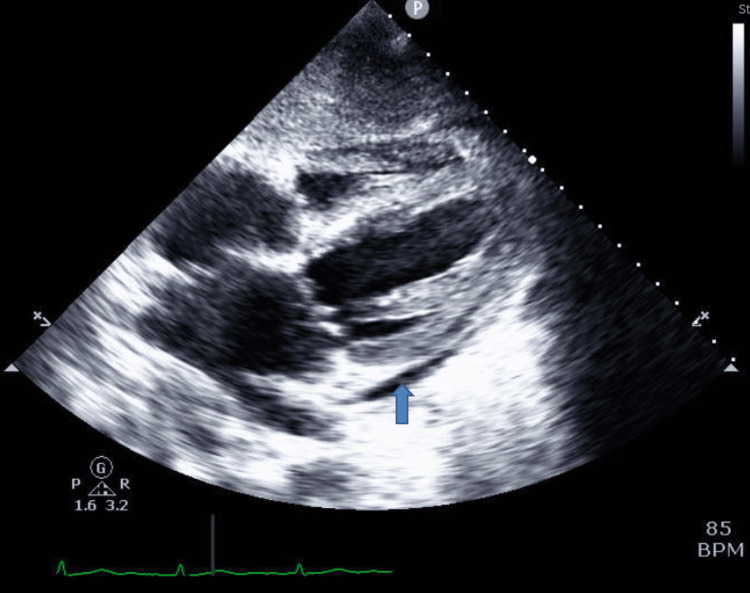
The initial transthoracic echocardiogram on admission shows a small pericardial effusion (blue arrow) on the parasternal long-axis view.
